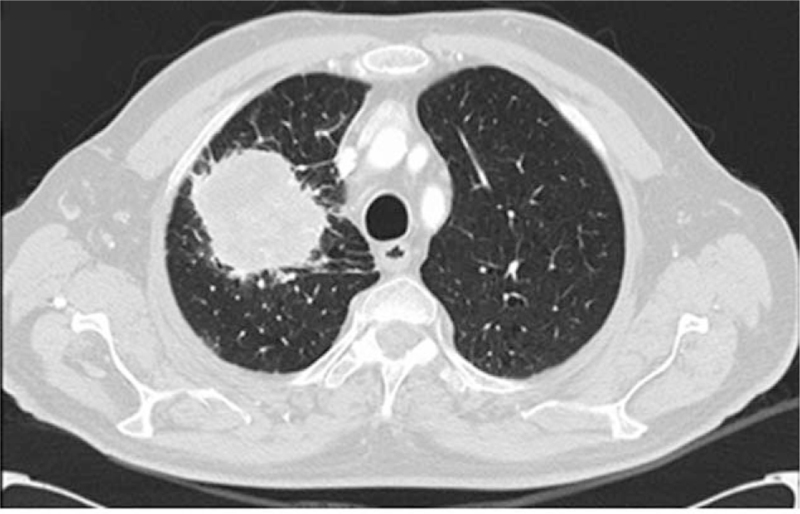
Computed tomography scan at diagnosis: right upper lung non-small cell lung carcinoma (NSCLC) (pT2bN0M0) of 6×6.5×4 cm.
